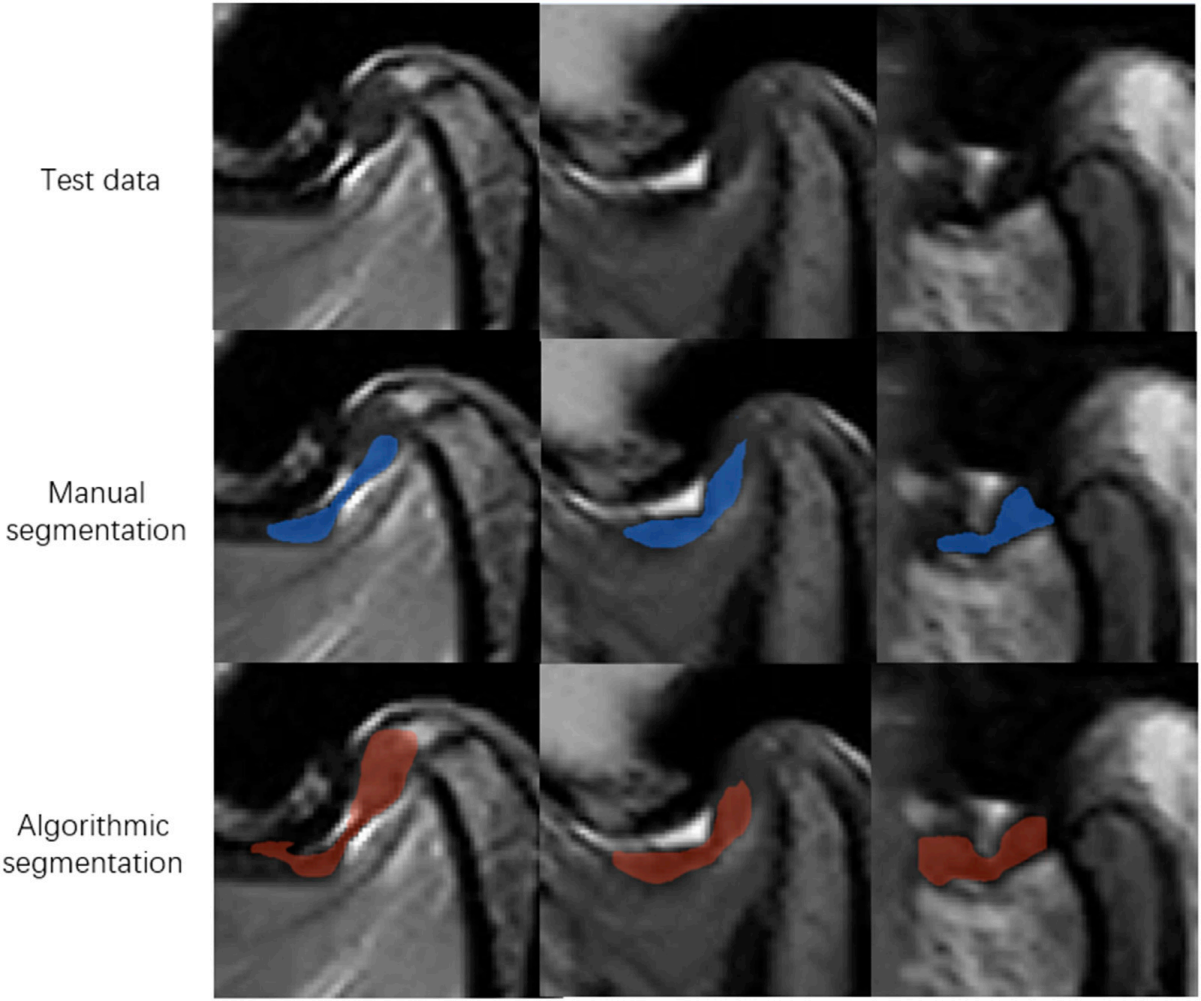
Representative images of model missegmentation. The first row shows the original MR images. The second row presents the manually segmented images by experts (blue regions). The third row depicts the images with model missegmentation (red regions).
